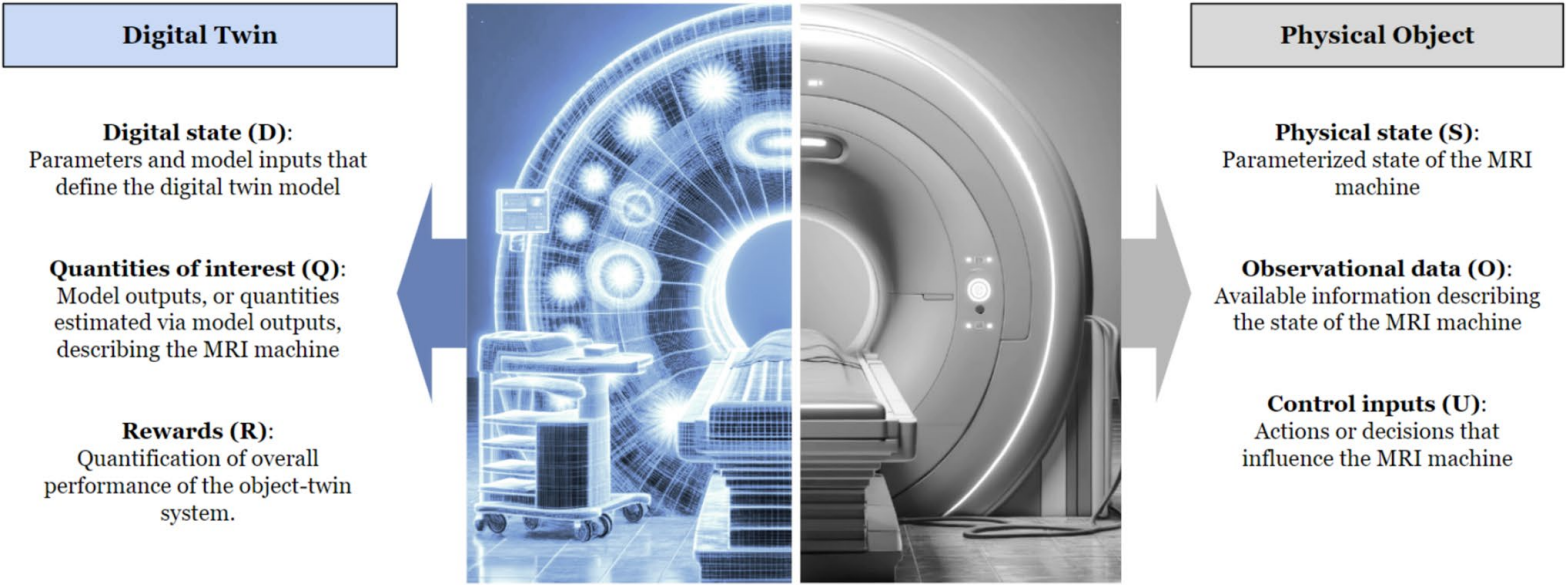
A mathematical abstraction of a digital twin and its associated MRI scanner
